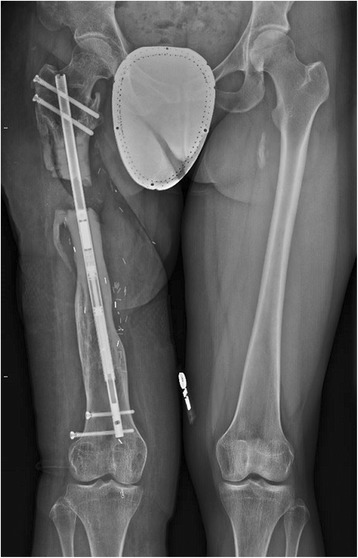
Long leg weight-bearing plain films demonstrating femur after four weeks of lengthening
